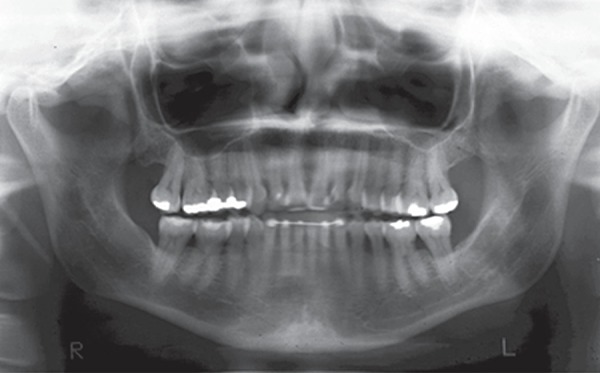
Follow-up panoramic radiograph
